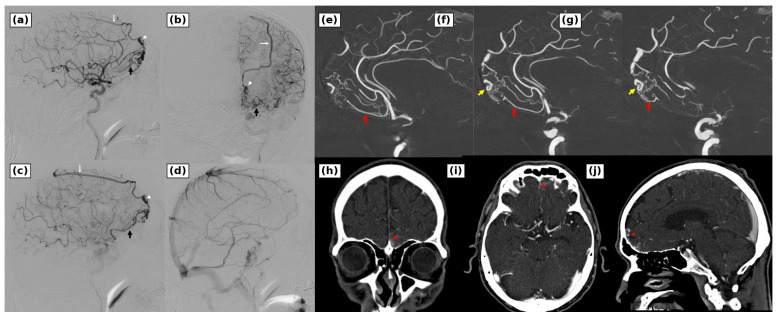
Diagnostic and surgical planning images of the anterior cranial fossa dural arteriovenous fistula. (a,b) Diagnostic left internal carotid artery angiography demonstrated a high-grade fistula at the left anterolateral cribriform plate, supplied predominantly by the left orbitofrontal artery with minor contributions from the right orbitofrontal artery and the left anterior ethmoidal artery. The fistula drained into the left ascending frontal vein and frontal bridging vein (white arrow) with a venous varix (white arrowheads), and also into the posterior fronto-orbital venous route (black arrow). (c,d) Later angiographic images demonstrated the lesion-related venous outflow and preserved normal venous drainage. (e–g) Three-dimensional rotational angiography delineated the steep orbitofrontal feeder arising from the anterior cerebral artery (red arrow), the shunt point (yellow arrow), cortical venous reflux, the venous varix, and the posterior fronto-orbital venous outflow toward the lateral cavernous sinus and pterygoid plexus. (h–j) Computed tomography angiography localized the fistulous point to the left lateral cribriform plate (red arrowheads) and demonstrated its relationship to the frontal sinus.
